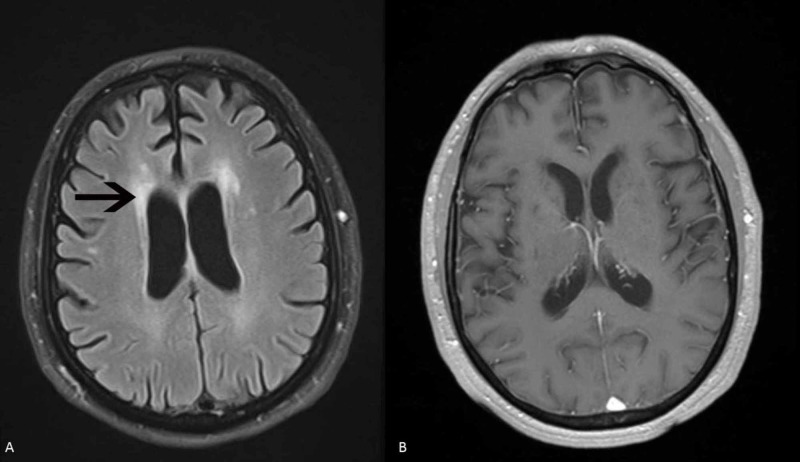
MRI of brain patient 1 (A) MRI of the brain FLAIR sequence shows mild ischemic gliotic changes bilaterally but no acute abnormalities. (B) T1 weighted image shows no enhancing lesions.
